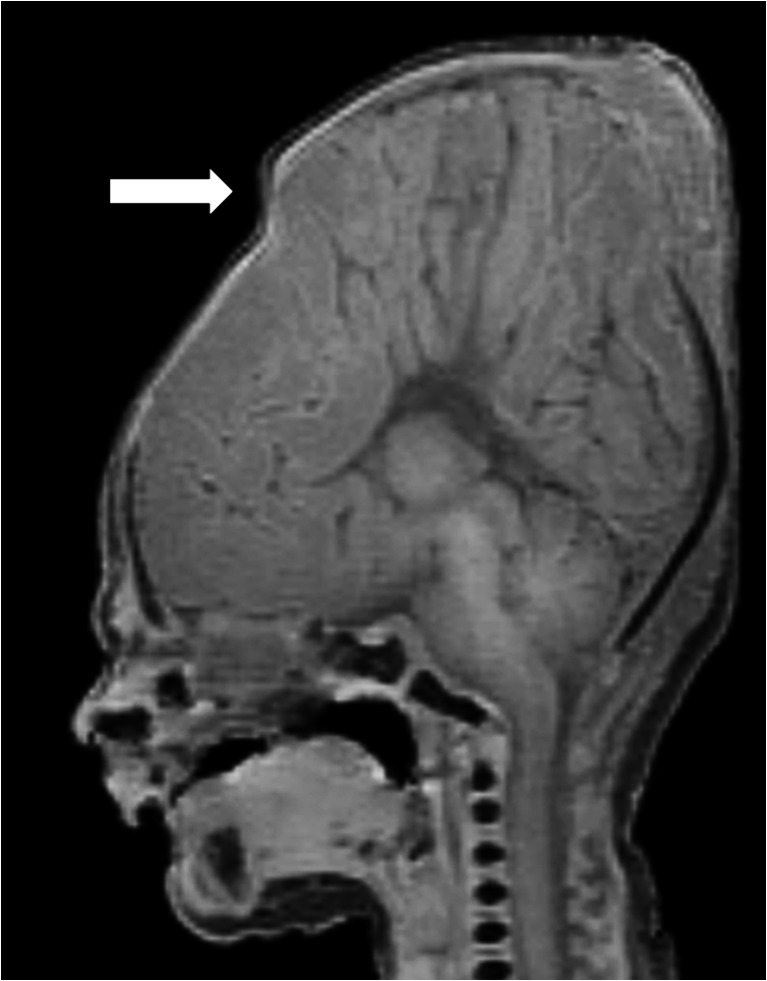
Head moulding. T1-weighted postmortem magnetic resonance imaging sagittal brain image of a 34-week gestation fetus with significant skull and head moulding, with separation of the sutures (*white arrow*) likely secondary to delivery. There was agenesis of the corpus callosum, confirmed at autopsy
